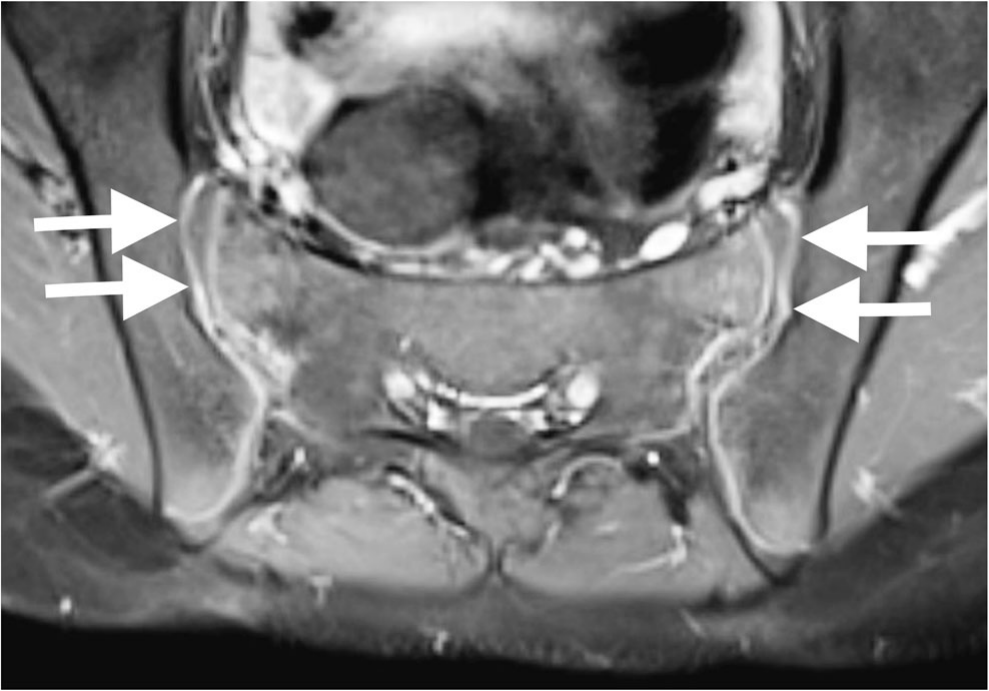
A 12-year-old girl with mechanical back pain. a Contrast-enhanced T1-weighted image (TR 679 ms, TE 12 ms) demonstrates smooth synovial enhancement (*arrows*) of both sacroiliac joints in the absence of other findings. The authors believe this may be a normal finding
